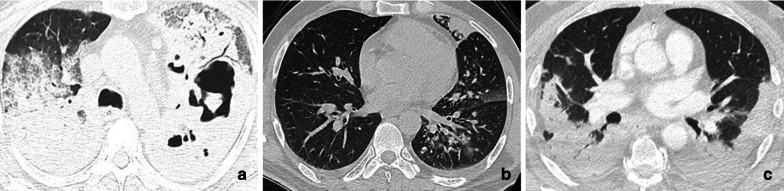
Atypical features for COVID-19. a A 47-year-old man with respiratory syncytial virus (RSV) pneumonia, axial HRCT image shows left pulmonary tree-in-bud opacities with centrilobular nodules, nearby patches of GGO and segmental consolidation anteriorly. b A 58-year-old woman with methicillin-resistant staphylococcus aureus (MRSA) pneumonia, HRCT shows bilateral consolidation with areas of GGO superimposed with crazy paving and cavitation on the left side. c A 67-year-old woman admitted to ICU with positive RT-PCR for COVID-19, axial HRCT show bilateral consolidation without significant GGO that was suggestive of coexisted bacterial infection. The causative organism of secondary infection was proved to be staphylococcus aureus, which is one of the commonest causes of hospital acquired infection
